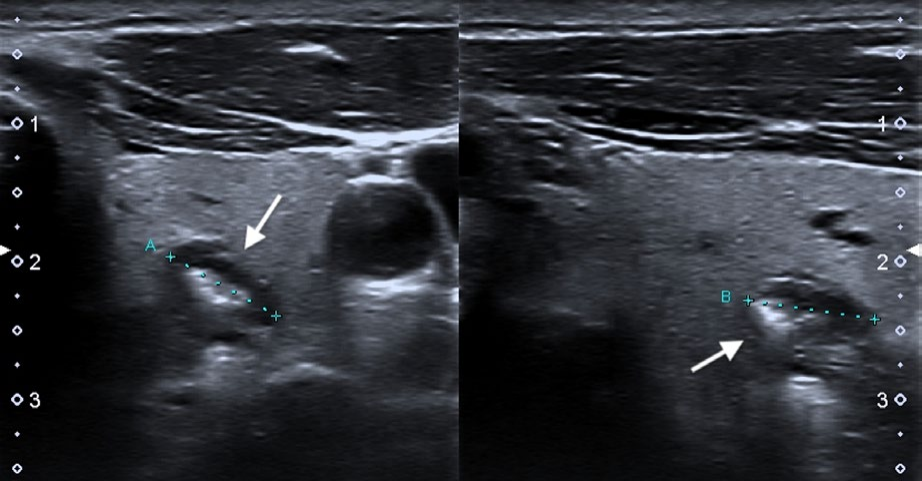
Thyroid ultrasound examination showed a hypoechoic nodule with bright internal hyperechoic foci and a partial surrounding halo involving the posterior aspect of the left thyroid lobe (white arrow)
